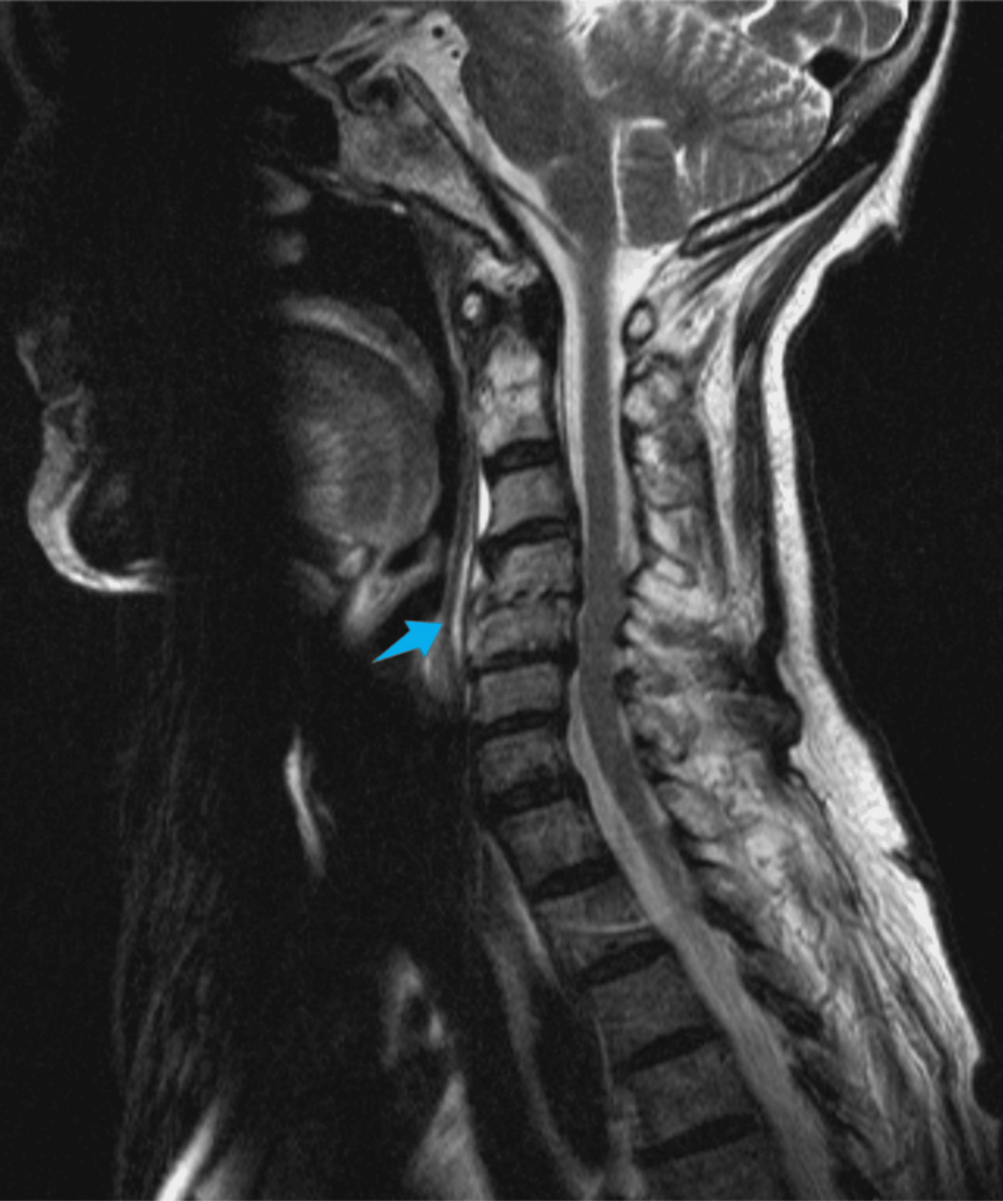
Sagittal T2-weighted MRI (August 2022) Compared to the previous MRI performed in June, this follow-up imaging demonstrates global improvement. The findings show a reduction in the height of the C4-C5 intervertebral disc and slight irregularities of the vertebral platforms with discreet signal reinforcement of these and the disc after contrast (arrow); marked reduction of the previously existing epidural component, currently only the presence of discrete signal reinforcement after contrast at the epidural level is identified, extending approximately from the plane of C3 to the plane of the lower vertebral platform of C5; additionally, there is a reduction of the peri-vertebral component.
